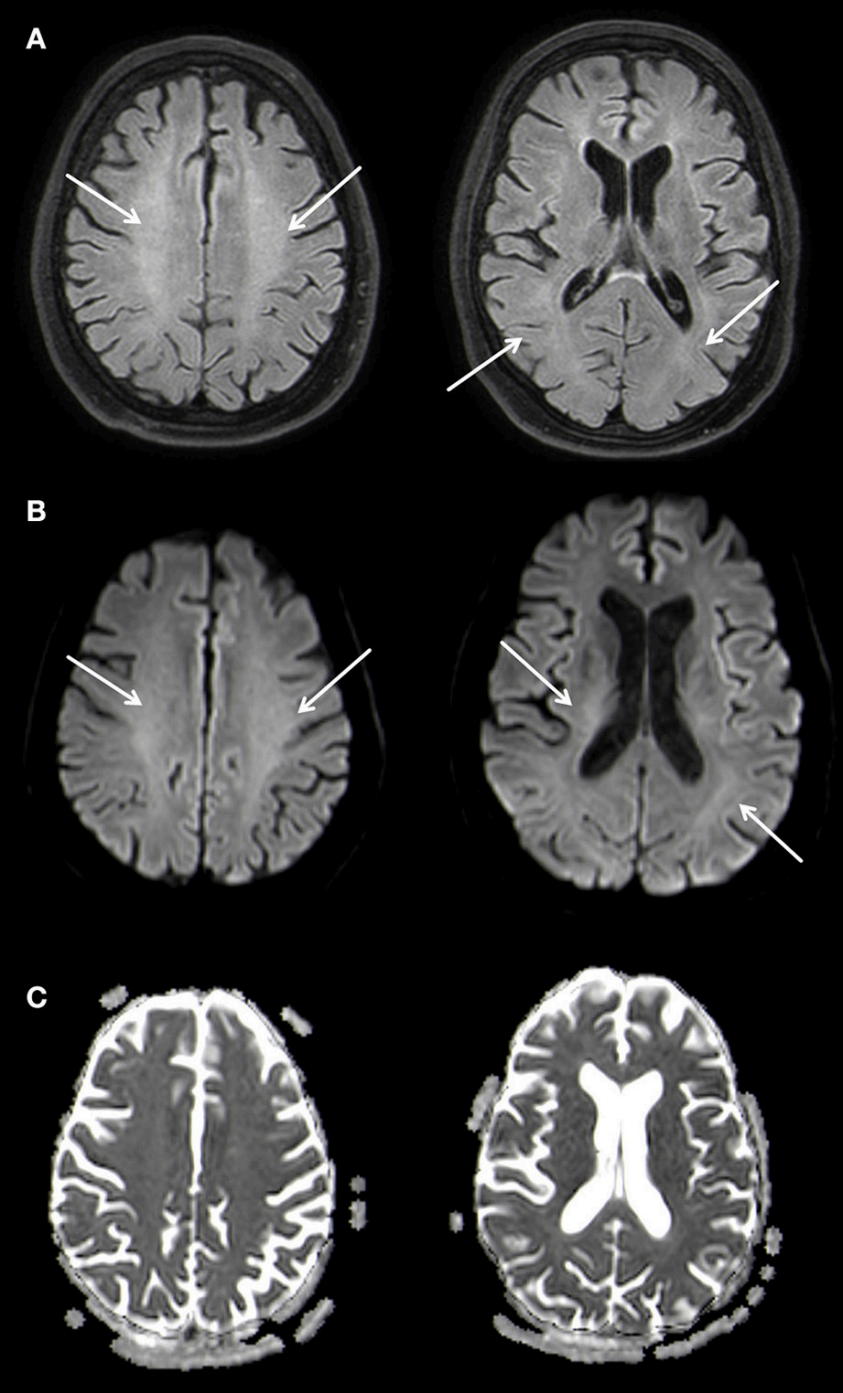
Slightly T2w hyperintense signal changes of the white matter (A) without diffusion restriction (B,C), Atophy of the parenchyma with slightly wider inner CSF. 3 T Philips Ingenia, (A) FLAIR, (B) b1000 Figure, (C) ADC Map. Overall there is a marked regression of the imaging findings.
